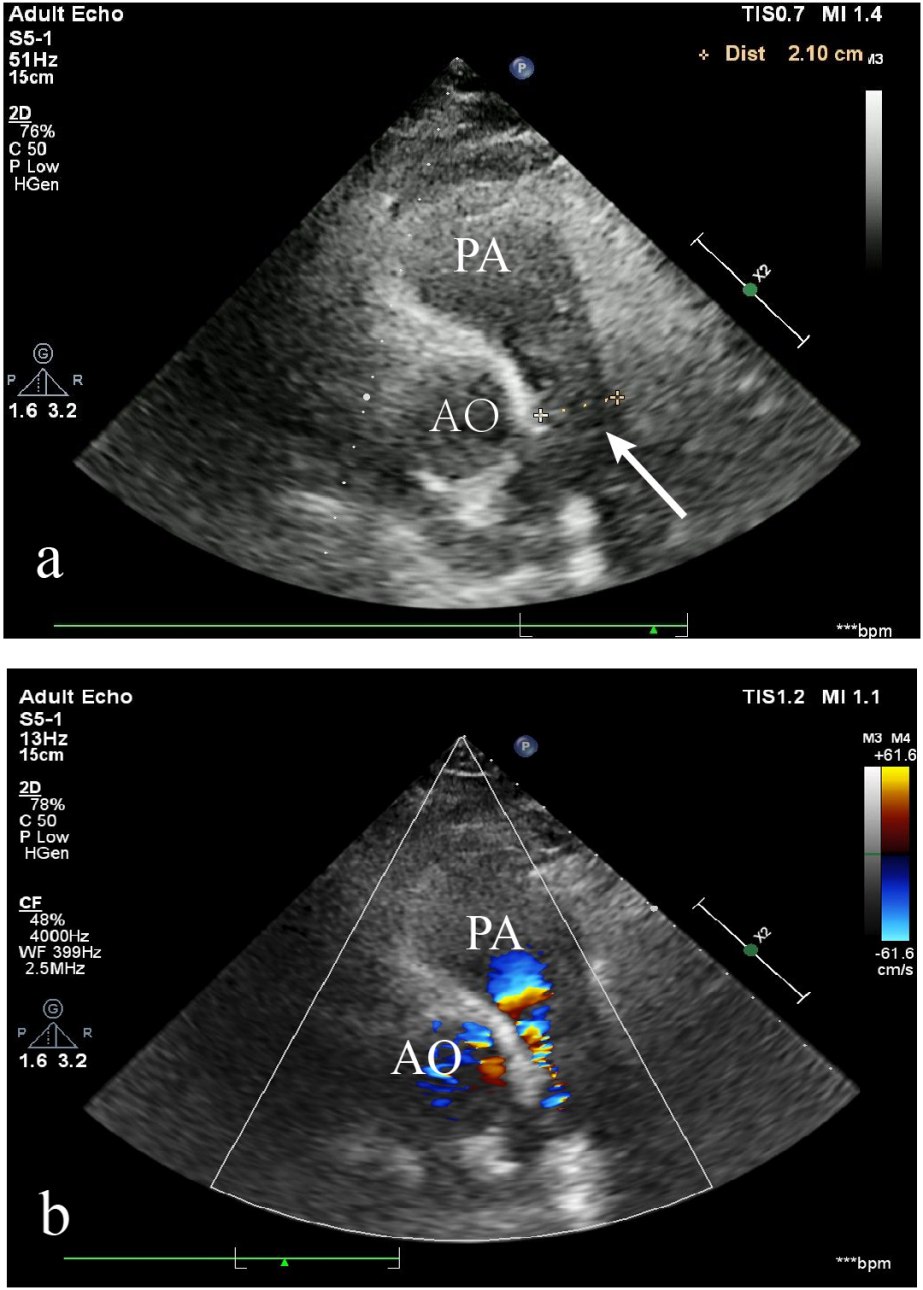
Transthoracic echocardiogram (parasternal short-axis view at the level of the great arteries). Abbreviations: AO, aorta; PA, pulmonary artery. (a) A hypoechoic mass (demarcated by white arrowheads) is visualized within the pulmonary artery, nearly occupying its entire lumen. (b) Color Doppler flow imaging demonstrates an eccentric, high-velocity, forward-flow jet across the obstruction within the narrowed residual lumen.
